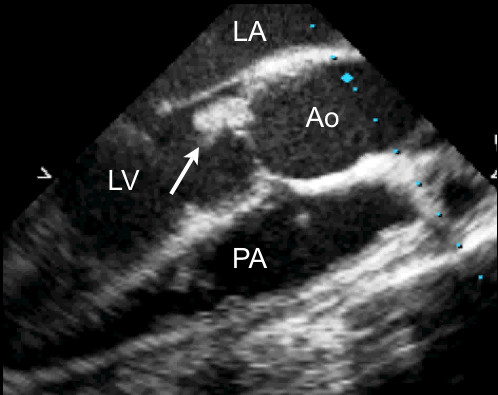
A transesophageal long axis view of the aorta in the same patient with demonstration of the vegetation on the noncoronary cusp of the aortic valve (arrow). LV, left ventricle; LA, left atrium; Ao; aorta; PA, pulmonary artery.
